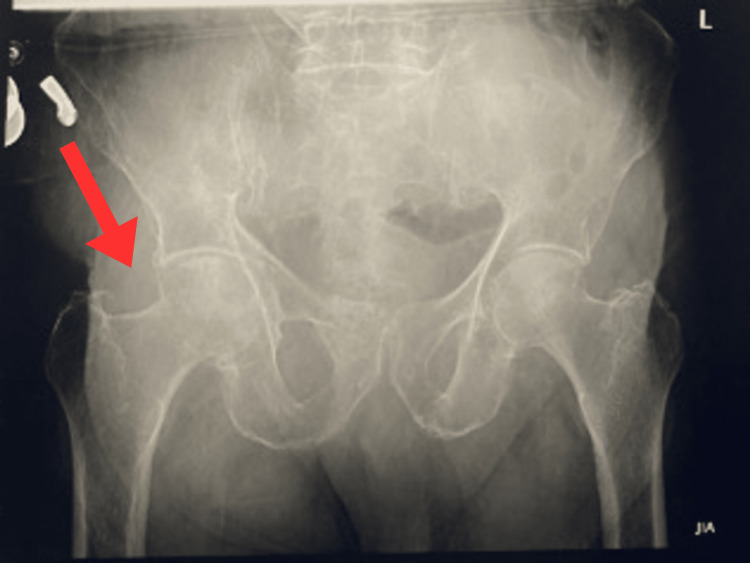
X-ray of the pelvis (AP view), 13 July 2018, showing normal proximal femoral anatomy prior to injury.
